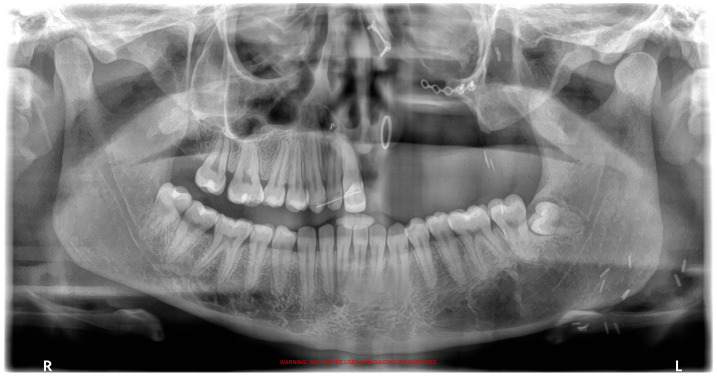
Panoramic radiograph after orthodontic treatment.
